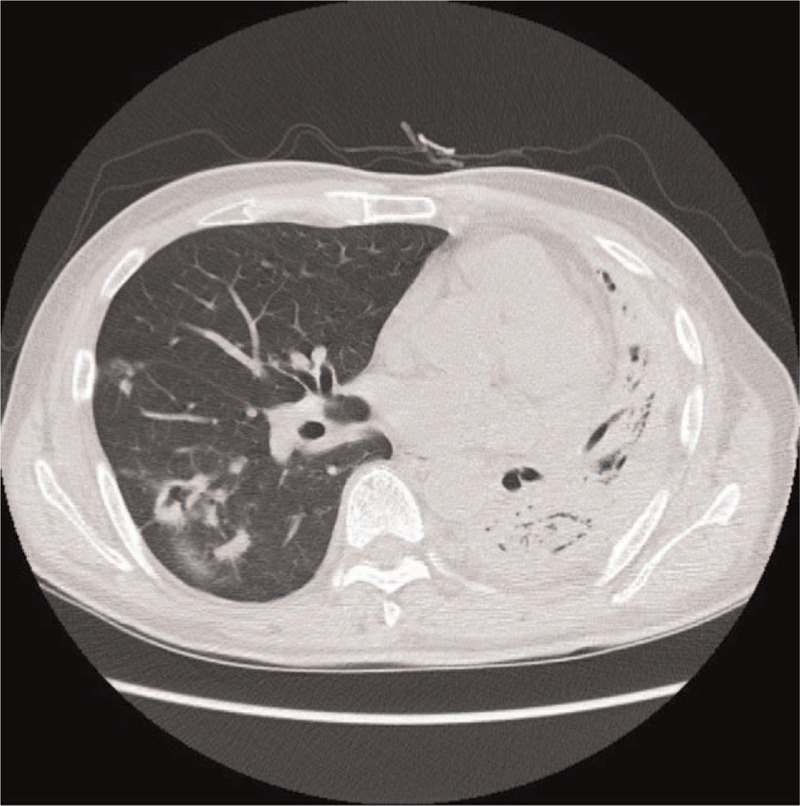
Preoperative chest computed tomography (axial view).
